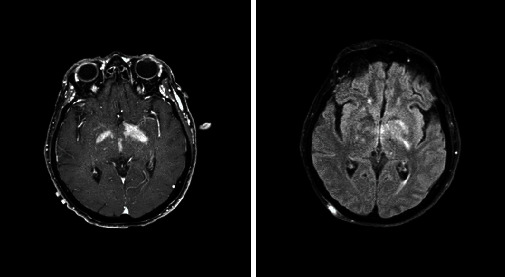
Enhancing lesions involving the bilateral cerebellar peduncles (right greater than left) as well as the bilateral internal capsules (left greater than right and the left globus pallidus). Radiographic differential diagnosis: infiltrating glioma and PCNSL.
